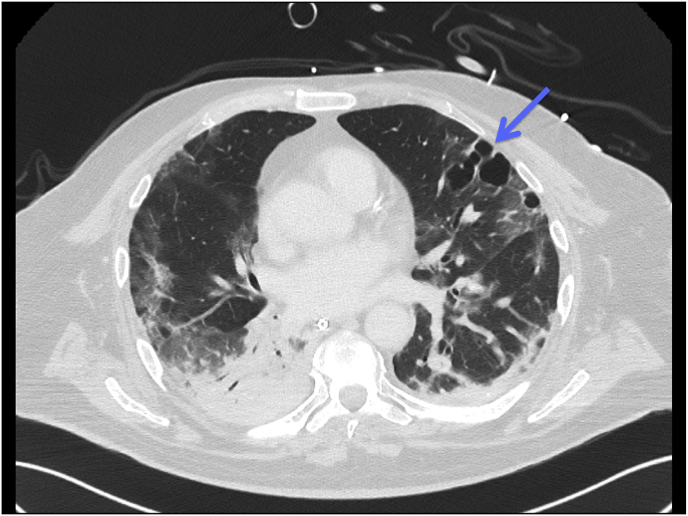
CT scan (day 28) demonstrating a large septated cavity in the lung parenchyma suspected to be an *Aspergillus* lesion (blue arrow). (For interpretation of the references to colour in this figure legend, the reader is referred to the Web version of this article.)
